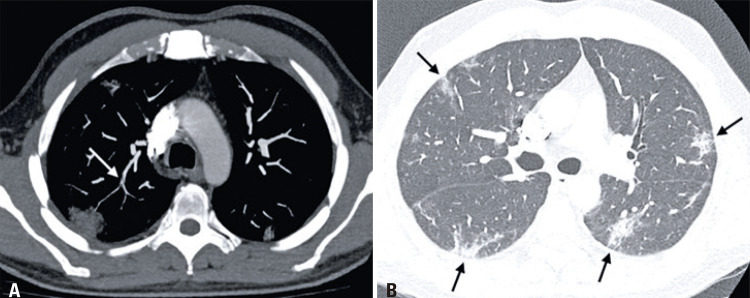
Computed tomography pulmonary angiography (49-year-old patient). (A) Axial mediastinal window shows filling defects of segmental and subsegmental artery branches of the right superior lobe (white arrows), findings compatible with pulmonary embolism. (B) Axial pulmonary window reveals multiple ground-glass opacities and thickened interlobular septae with bilateral and peripheral distribution (black arrows), findings consistent with coronavirus pneumonia
